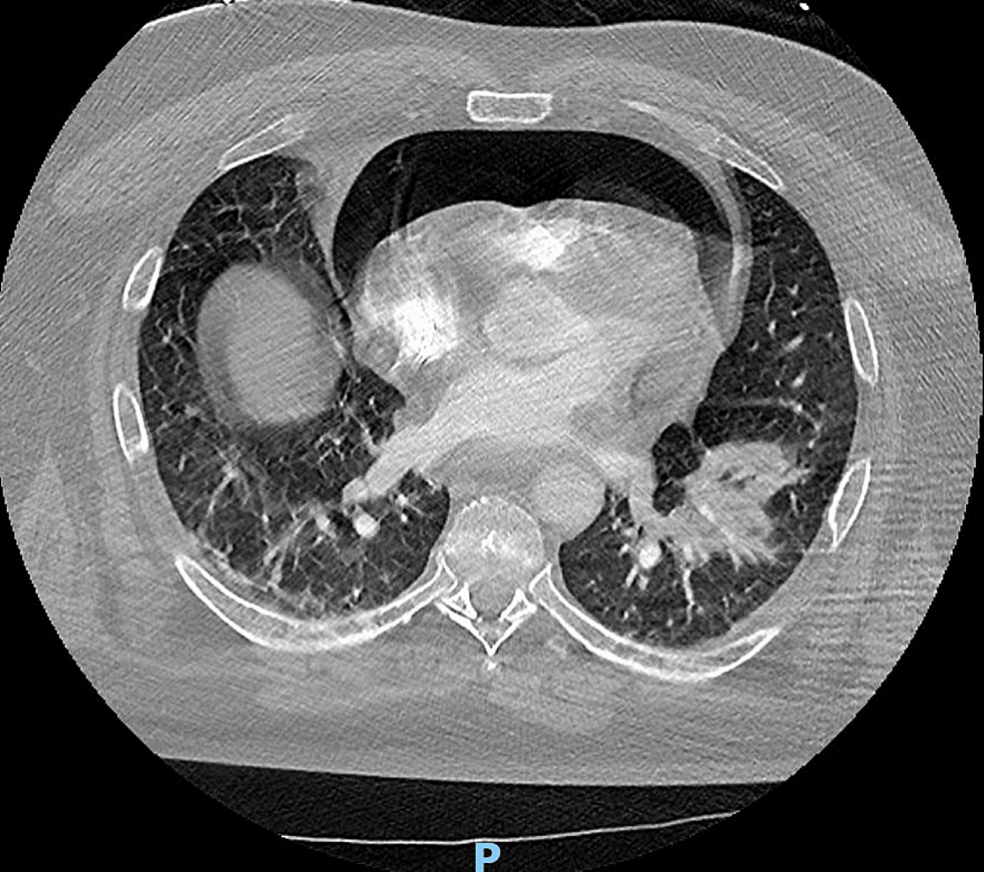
CT scan showing air around the heart (pneumopericardium)
